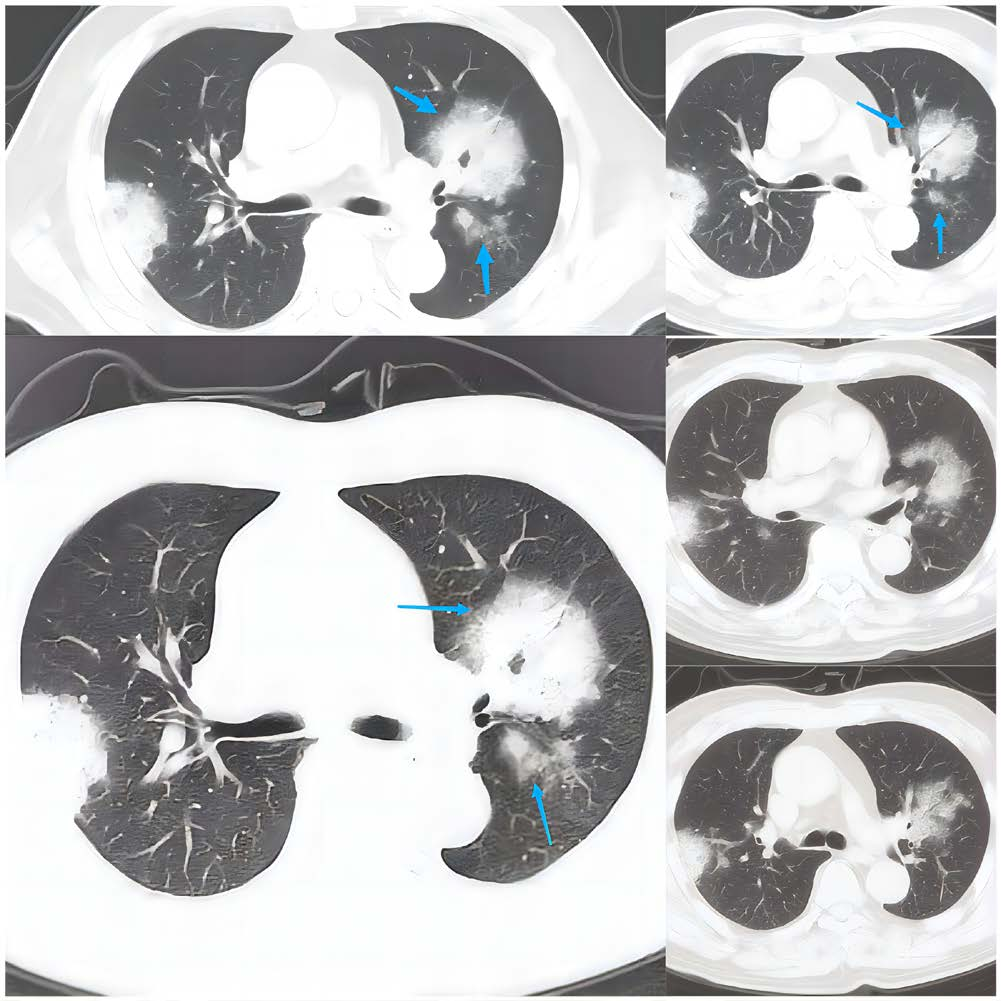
Displays the patient’s complete lung CT scan. The presence of chest tightness and shortness of breath indicated potential infectious lesions in both lungs, with faint shadows observed around the left lung, as indicated by the arrows.
